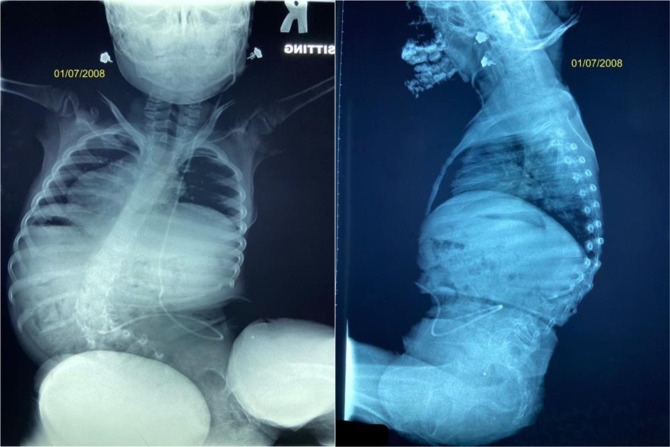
Radiograph showing a six-year-old female patient with a thoracic level nonambulatory myelomeningocele.
